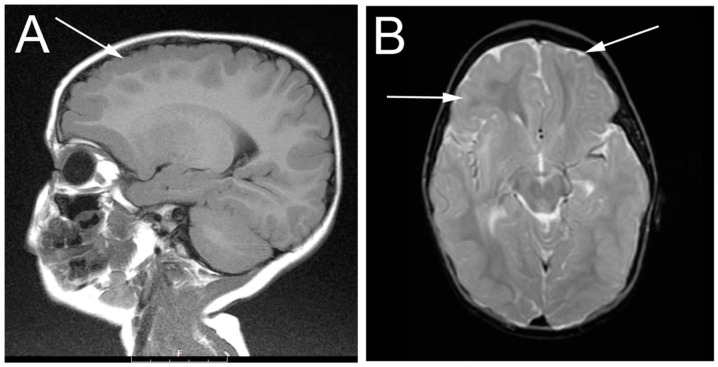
Magnetic resonance imaging (MRI) imaging of the patient at two years of age showing frontal lobe bilateral polymicrogyria (highlighted by arrows). (A) sagittal plane; (B) transverse plane.
